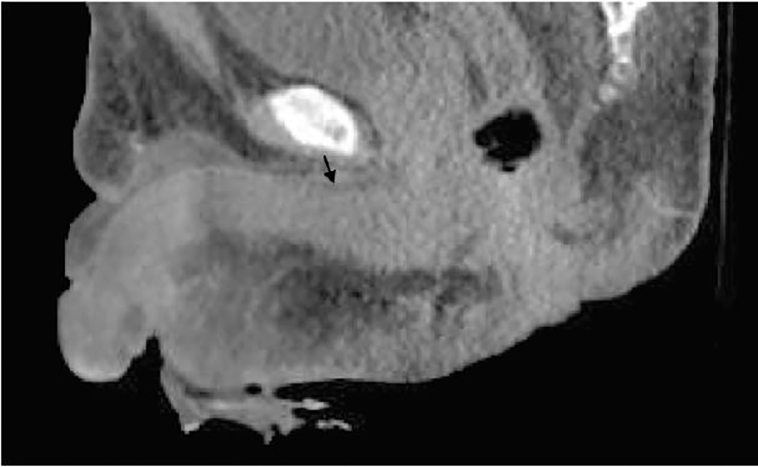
Computed tomography (sagittal view) of genitalia showing focal fluid 1.0 cm in thickness tracking along the superior aspect of the penile shaft (black arrow)
